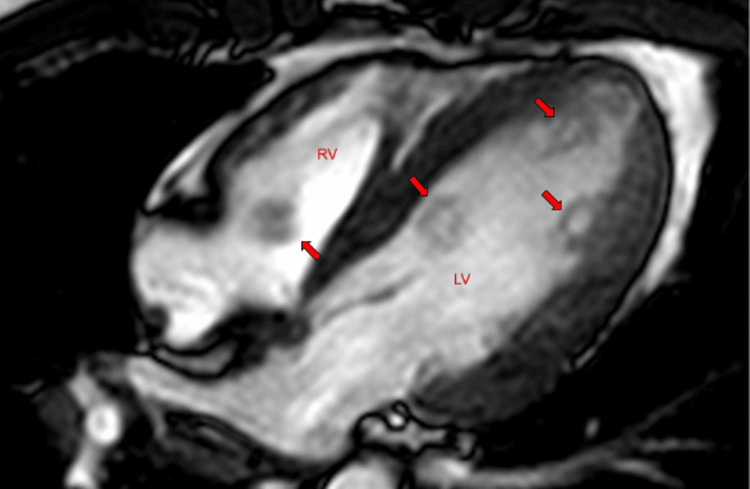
Cardiac MRI, left ventricular (LV) and right ventricular (RV) melanomas (arrows). Source: Ref. [8]
